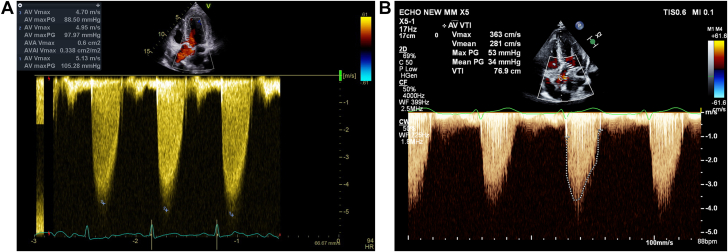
Echocardiogram: Continuous-Wave Doppler Imaging of the Aortic Valve (A) Before intervention. (B) After transcatheter aortic valve replacement. AV = aortic valve; CF = color flow; CW = continuous wave; ECHO = echocardiogram; max = maximum; min = minimum; PG = pressure gradient; V = velocity; VTI = velocity time integral; 2D = 2-dimensional.
